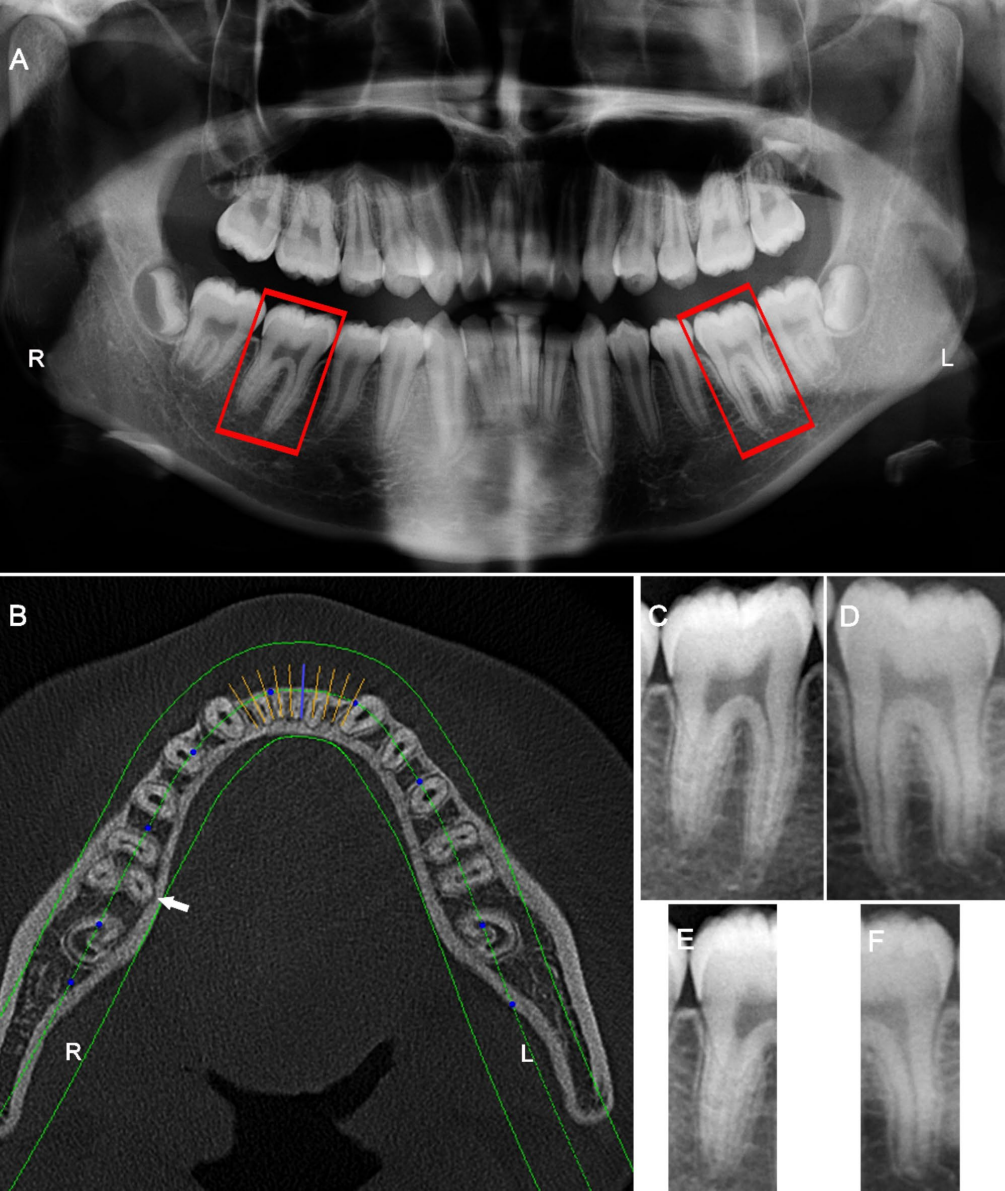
Preparation of image patches of mandibular first molars (MFMs) (The CBCT and panoramic images were derived from a same patient). (A) A panoramic radiograph; (B) an axial CBCT cross-section shows a three-rooted MFM (white arrow) on the right side and a two-rooted MFM on the left side (the gold standard for labeling image patches); (C and D) image patches of the right and left MFMs were cropped from the panoramic radiograph; (E and F) panoramic image patches of the distal halves of bilateral MFMs.
